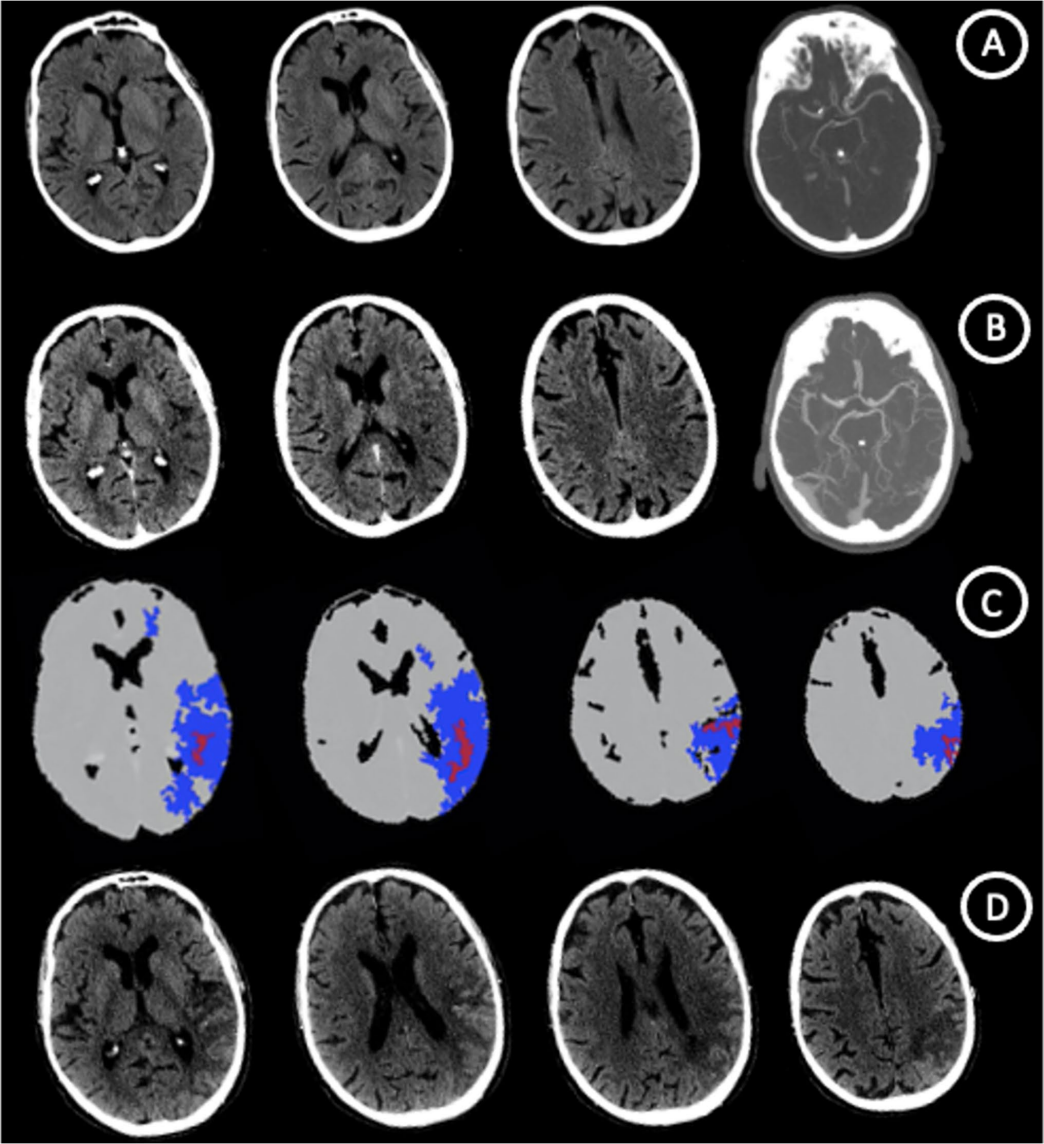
Neuroimaging study protocol in spoke and hub center: NCCT with ASPECT score of 6 and distal M1 cerebral artery occlusion. (A) NCCT, mCTA and CTP > 6 h from symptom onset with spontaneous recanalization and ischemic core underestimation due to luxury perfusion (B,C). NCCT at 3 days uphold a large ischemic core size (D)
